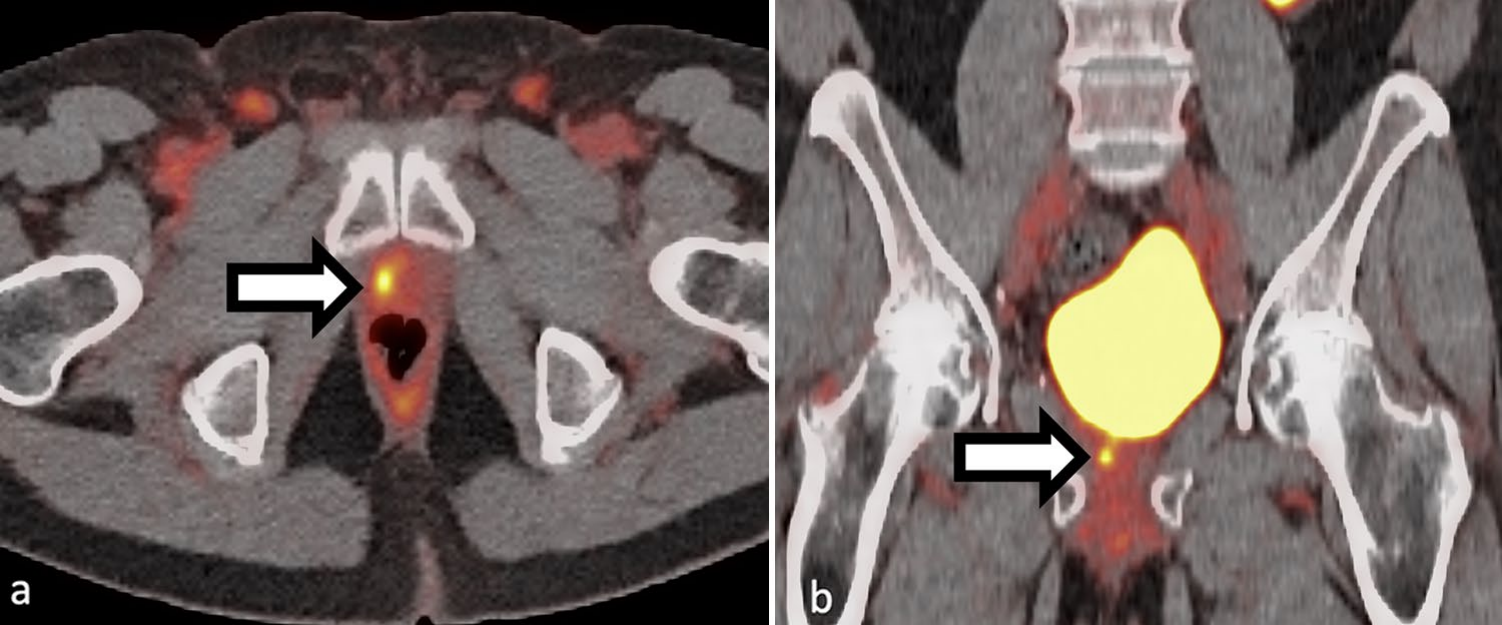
a–b Example of local recurrence in a 75-year-old patient presenting with slowly rising PSA after prostatectomy 20 years prior. F18-DCFPyL PET/CT shows focal uptake in the right surgical bed at the anastomosis (white arrows in panels a and b)
